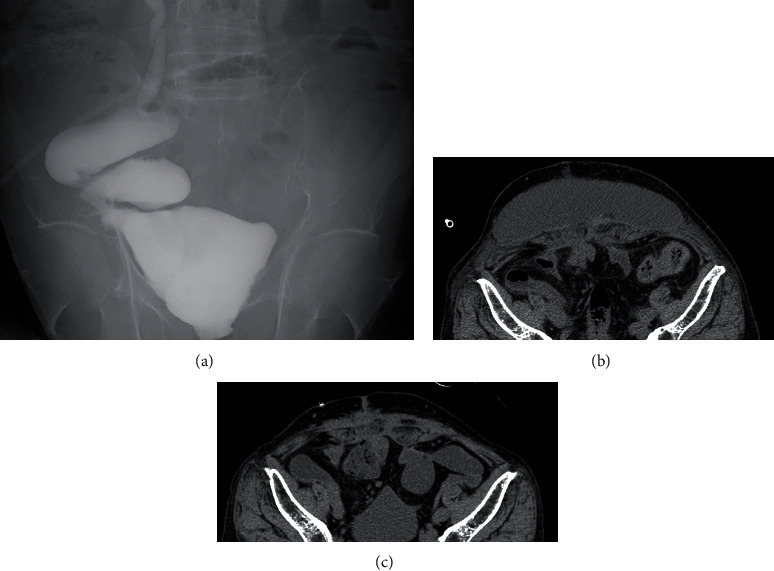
Postoperative cystography and computed tomography (CT) scan. Cystography of the neobladder showed no leakage (a). Subcutaneous fluid collection is observed on postoperative day 14 (b). It improved by postoperative day 29 (c).
